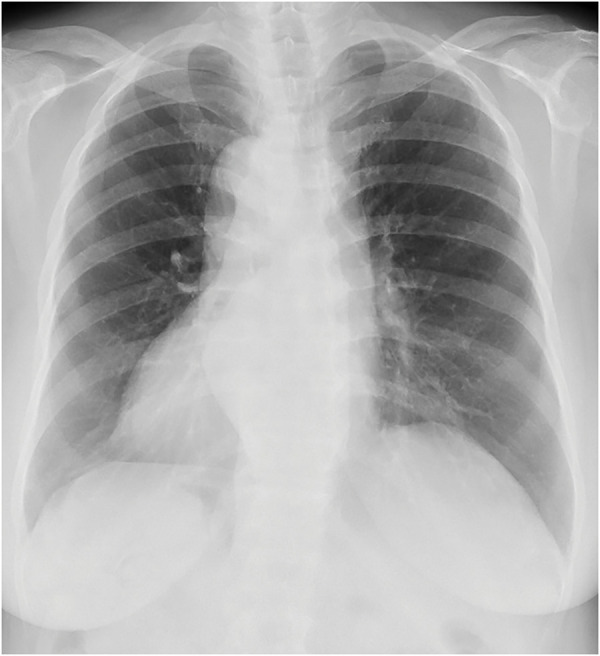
X-ray images of the patient. Dextrocardia is seen.
